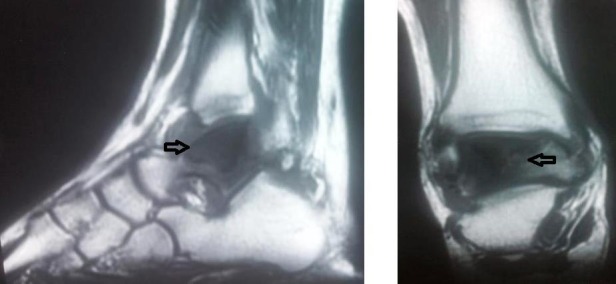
Magnetic resonance imaging (MRI) of the right ankle. Hypo-intense lesions in the talus bone on T1 weighted images (black arrows), consistent with an inflammatory focus in the talar bone.
